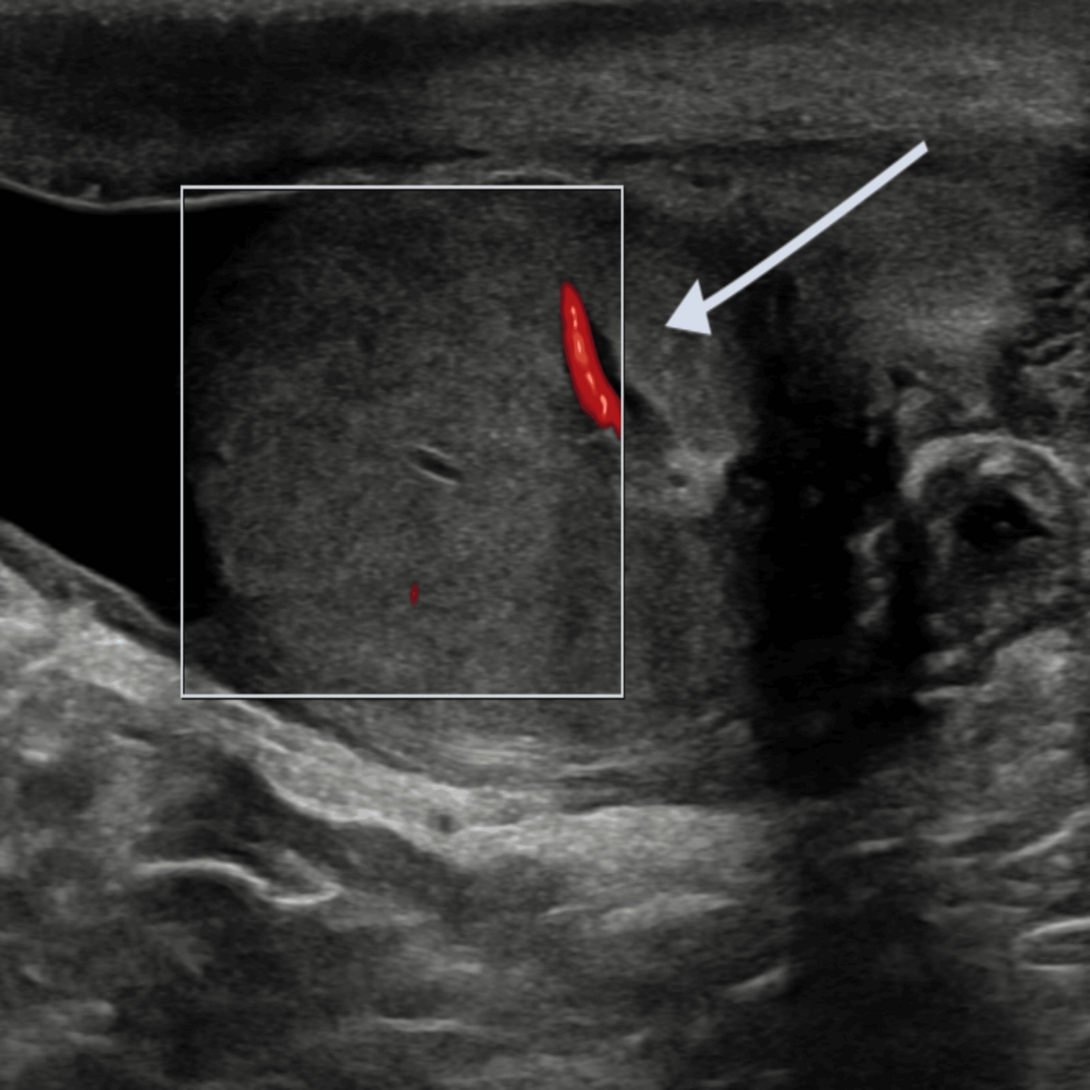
Color Doppler image demonstrating preserved testicular perfusion.
